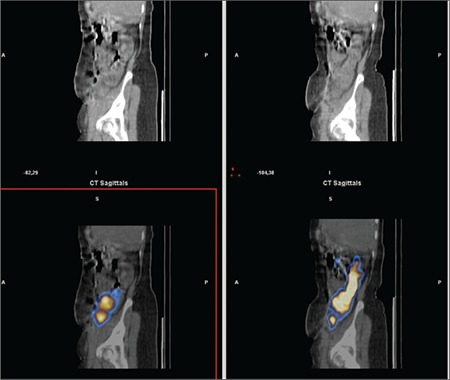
Comparison of early and late phase SPECT/CT images; saggital Slices
